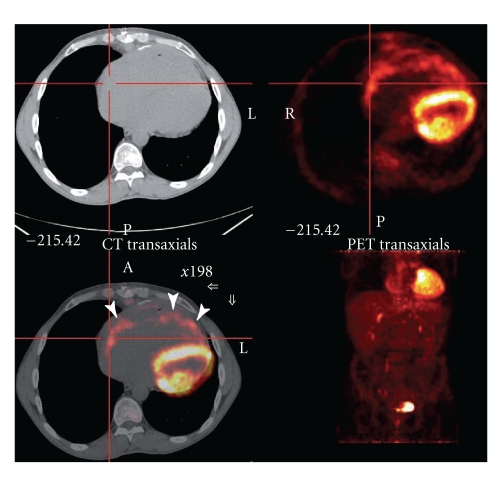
FDG-PET in the transaxial plane shows moderate FDG uptake (arrows) involving mainly the right heart.
